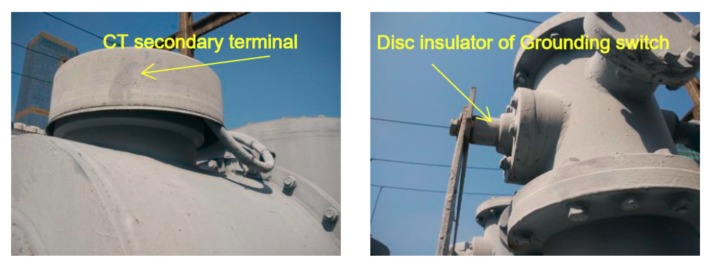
Appearance of CT secondary terminal and disc insulator of grounding switchgear.
